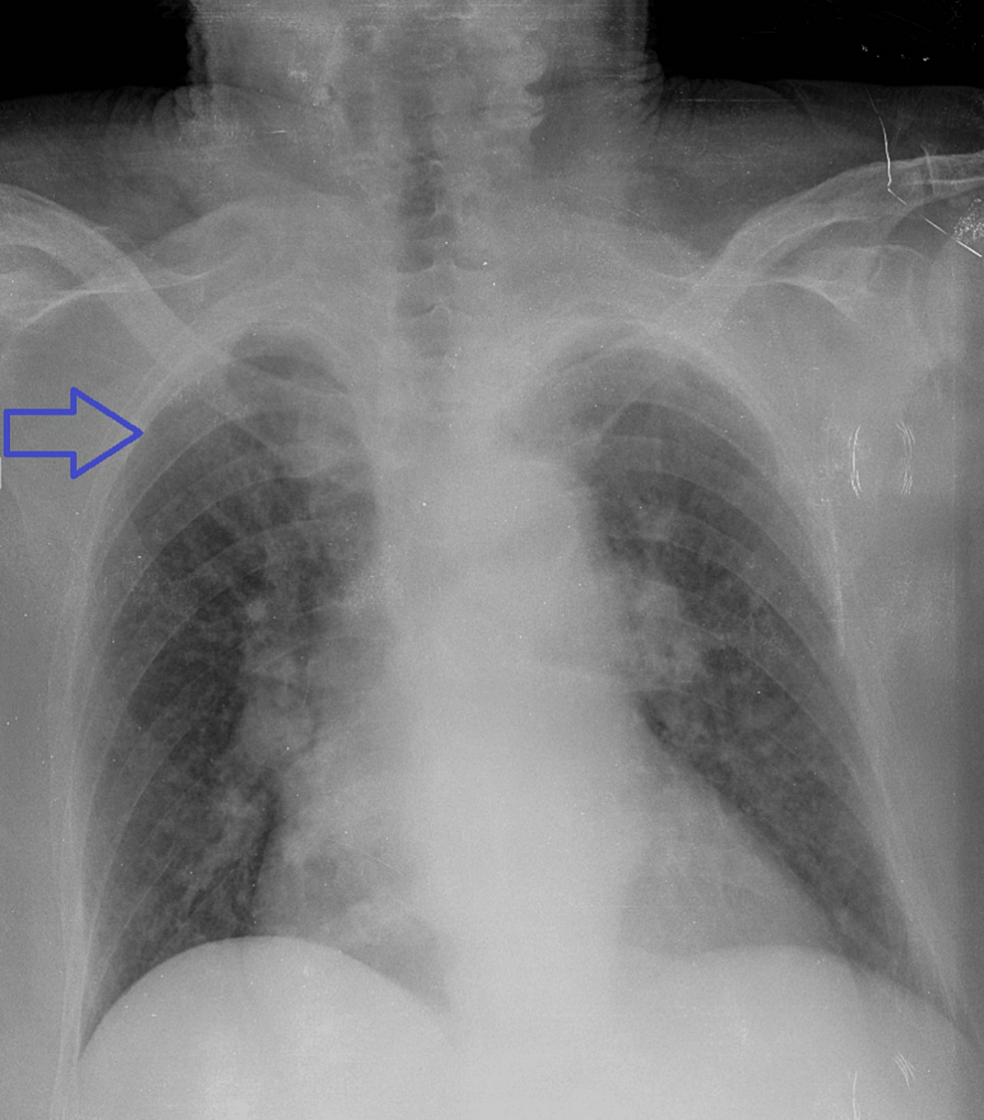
Chest X-ray displaying the area of diminished respiratory wheeze on the right upper pulmonary field (blue arrow)
